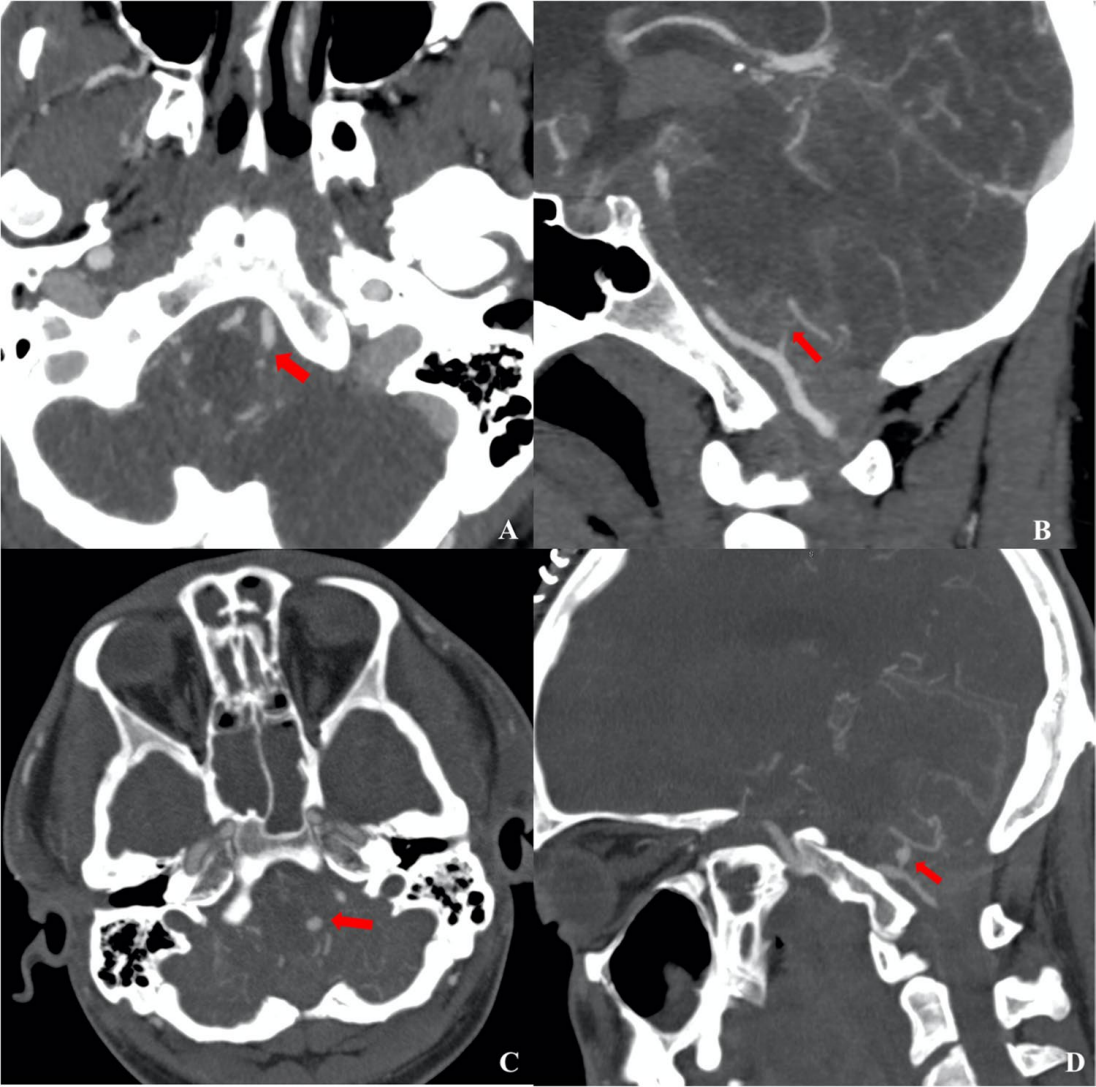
A Axial and B sagittal scans o the first angio-CT and the same scans in C and D 9 days after the trauma. The arrows highlight the site of the left PICA where the post-traumatic aneurysm has formed
